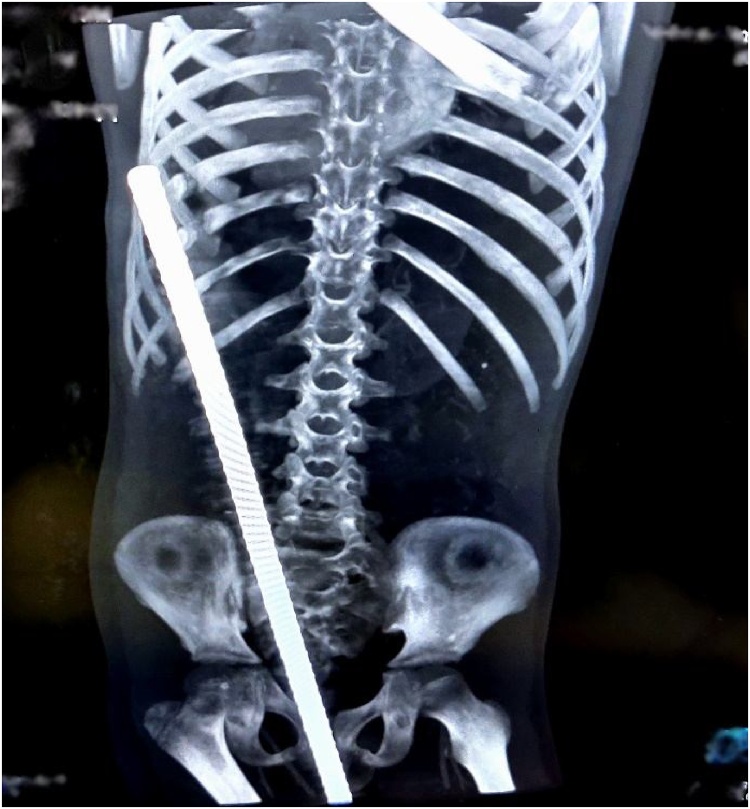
CT scan (coronal view) showing metallic foreign body (rod) extending from right side of perineum to left upper abdomen.
